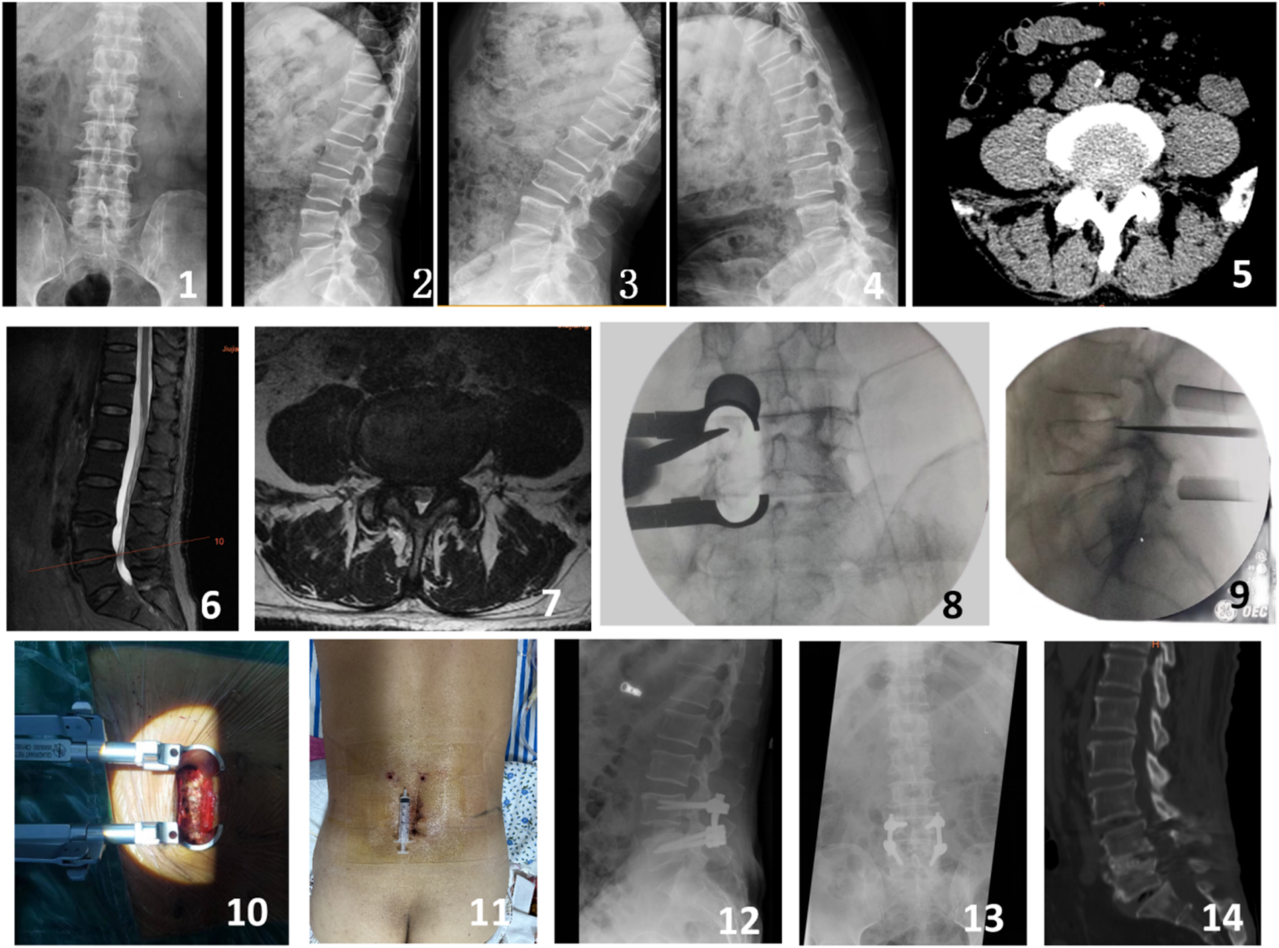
A 68-year-old male presented with intermittent claudication and numbness in the left lower limb for 4 years. Preoperative imaging included lateral, anteroposterior, and dynamic flexion-extension lumbar radiographs (Figures 1-4), preoperative CT scan (Figure 5), and preoperative MRI (Figures 6-7). Intraoperative images captured the process of the modified single-incision surgery (Figures 8-10). The postoperative incision was sutured and measured approximately 5 cm (Figure 11). Postoperative imaging at 3 days following surgery included lateral and anteroposterior lumbar radiographs (Figures 12-13). A CT scan at 12 months postoperatively showed good bony fusion (Figure 14).
